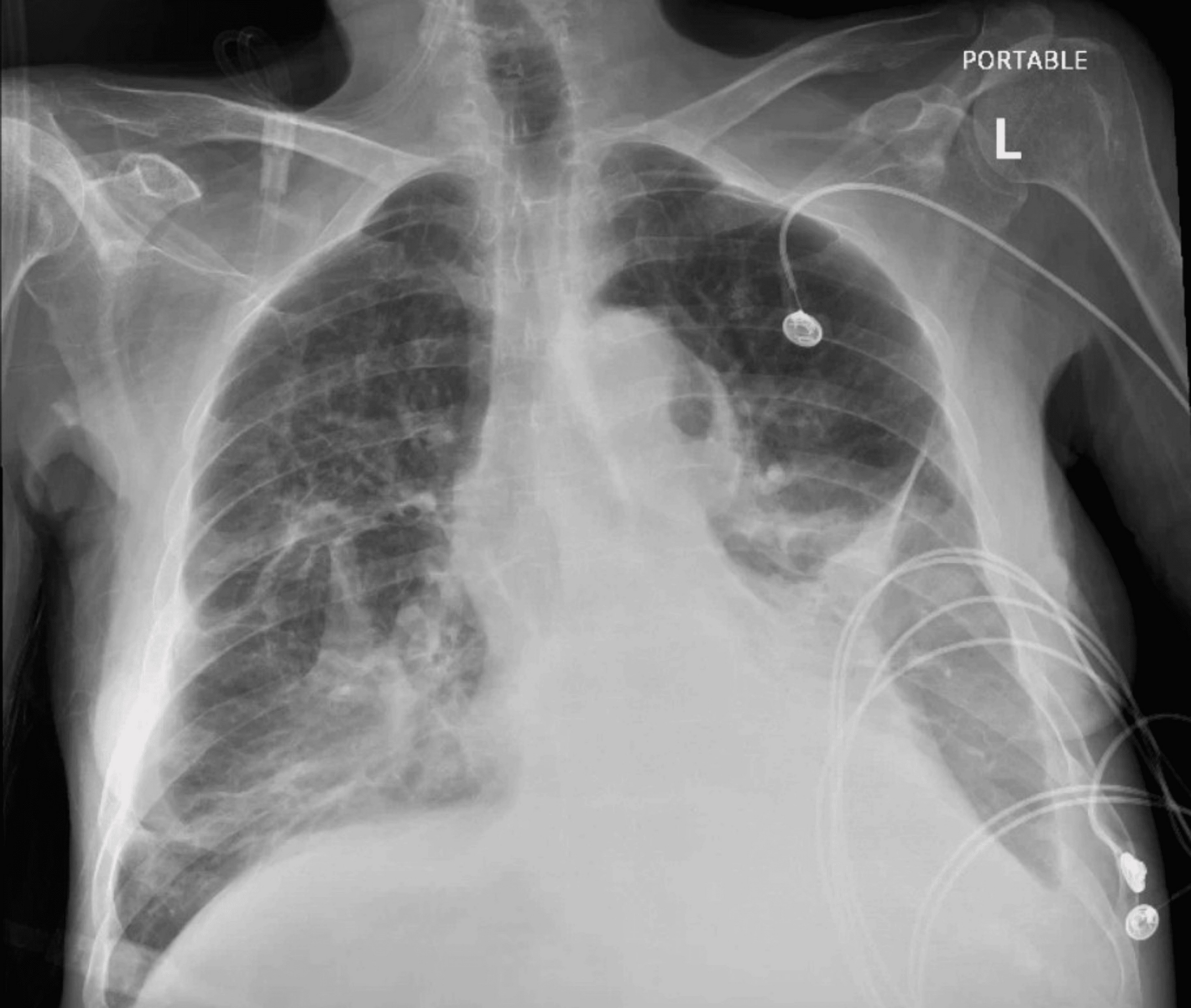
X-ray chest demonstrating bilateral predominantly lower zone opacities
